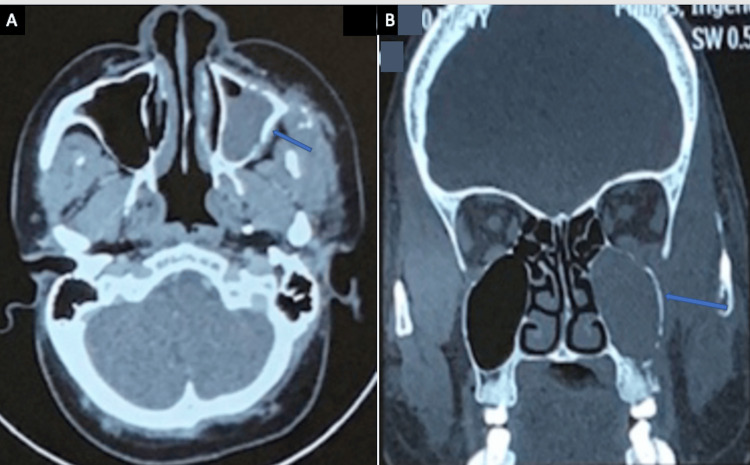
(A) A case of acute invasive sinonasal fungal infection showing left maxillary sinus infiltration (↗) (CT scan, axial). (B) CT scan coronal bone window highlighting left posterior maxillary wall erosion (↗) (CT scan, coronal bone window). CT: computed tomography
